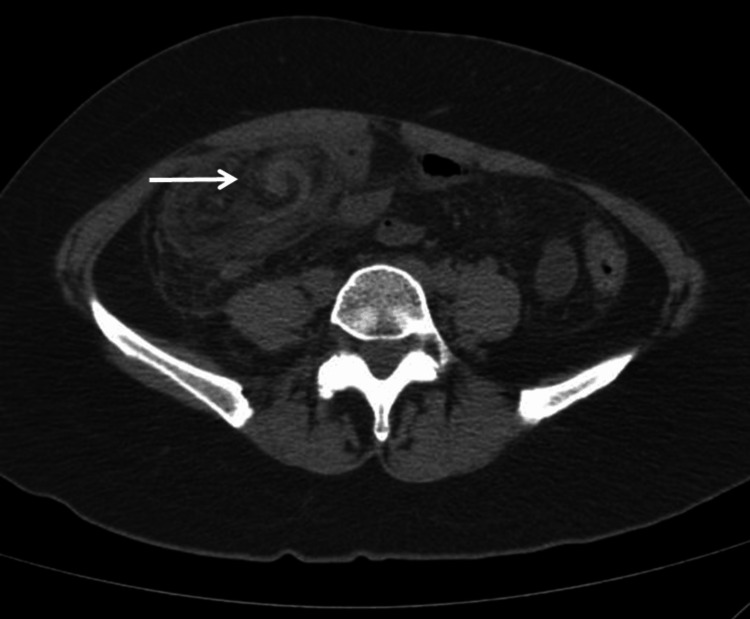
Computerized tomography in a simple phase of the abdomen. An axial CT scan shows elongated structures (blood vessels) within the mass forming a concentric circle pattern, characteristic of the whirl sign (thin arrow).
